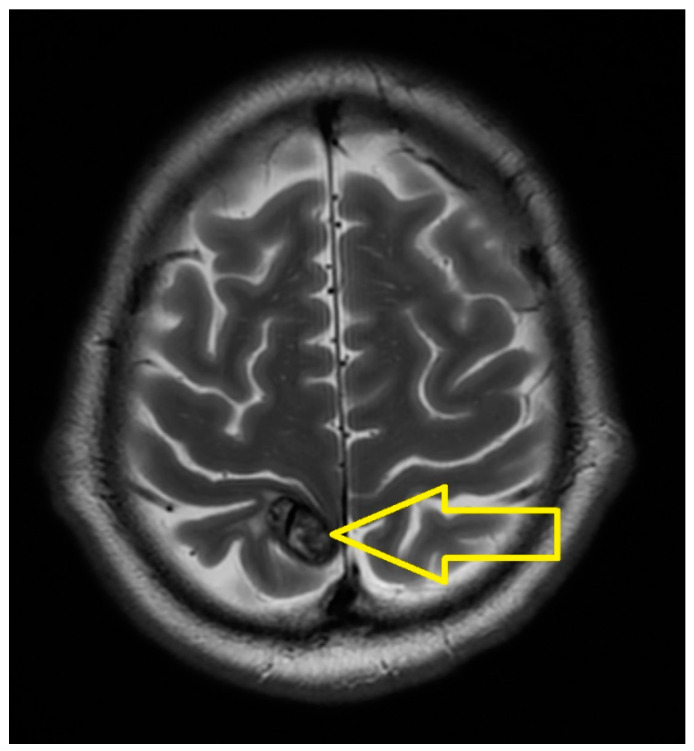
Cerebral MRI (June 2023): Dimensional regression of the right parietal tumor mass and of the perilesional edema.
